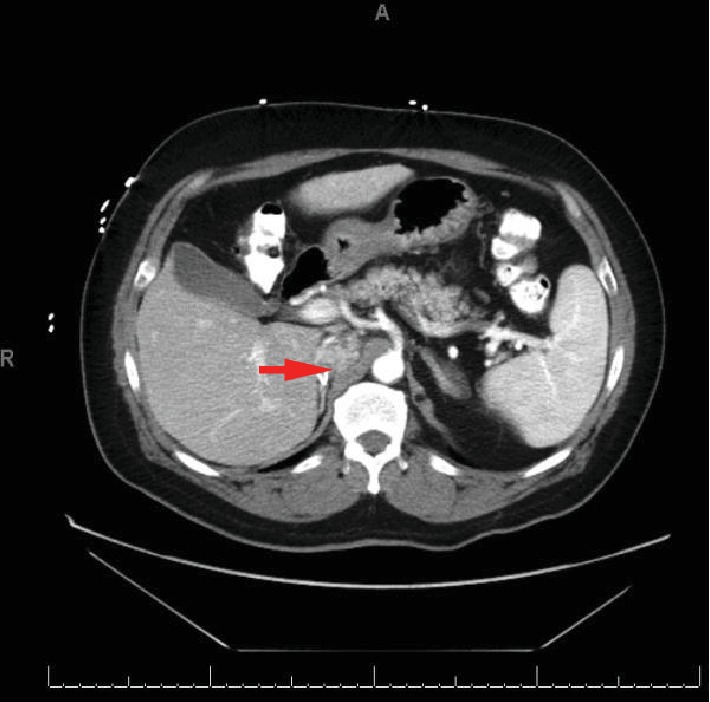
CT abdomen and pelvis w/ contrast; arrow showing heterogenous density 2.5 × 3.5 cm lesion in vicinity of the right nephrectomy bed compressing the inferior vena cava.
